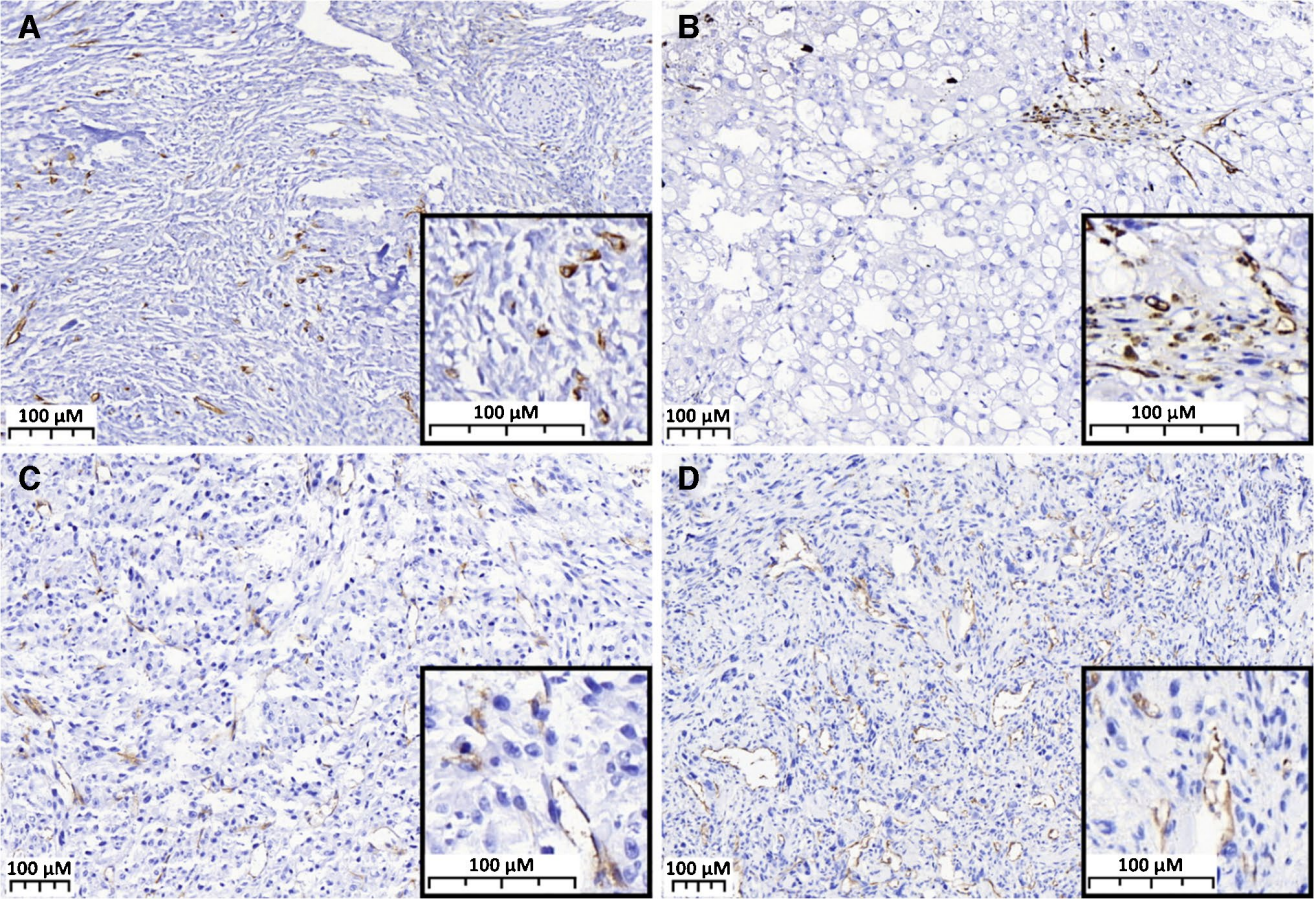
PSMA expression in the neovasculature of bone tumors. A ABC showing intense and diffuse expression of PSMA. B Chordoma showing intense and diffuse expression of PSMA. C Dedifferentiated chondrosarcoma with PSMA index 1 in the dedifferentiated component. D Osteosarcoma with PSMA index 2
